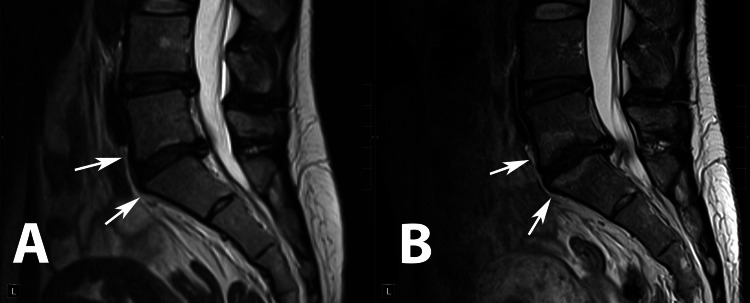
Sagittal T2-weighted MRI images of Patient 2 at initial presentation (A) and three years later. Development of Modic changes in L5, S1 vertebral end plates (white arrows).
